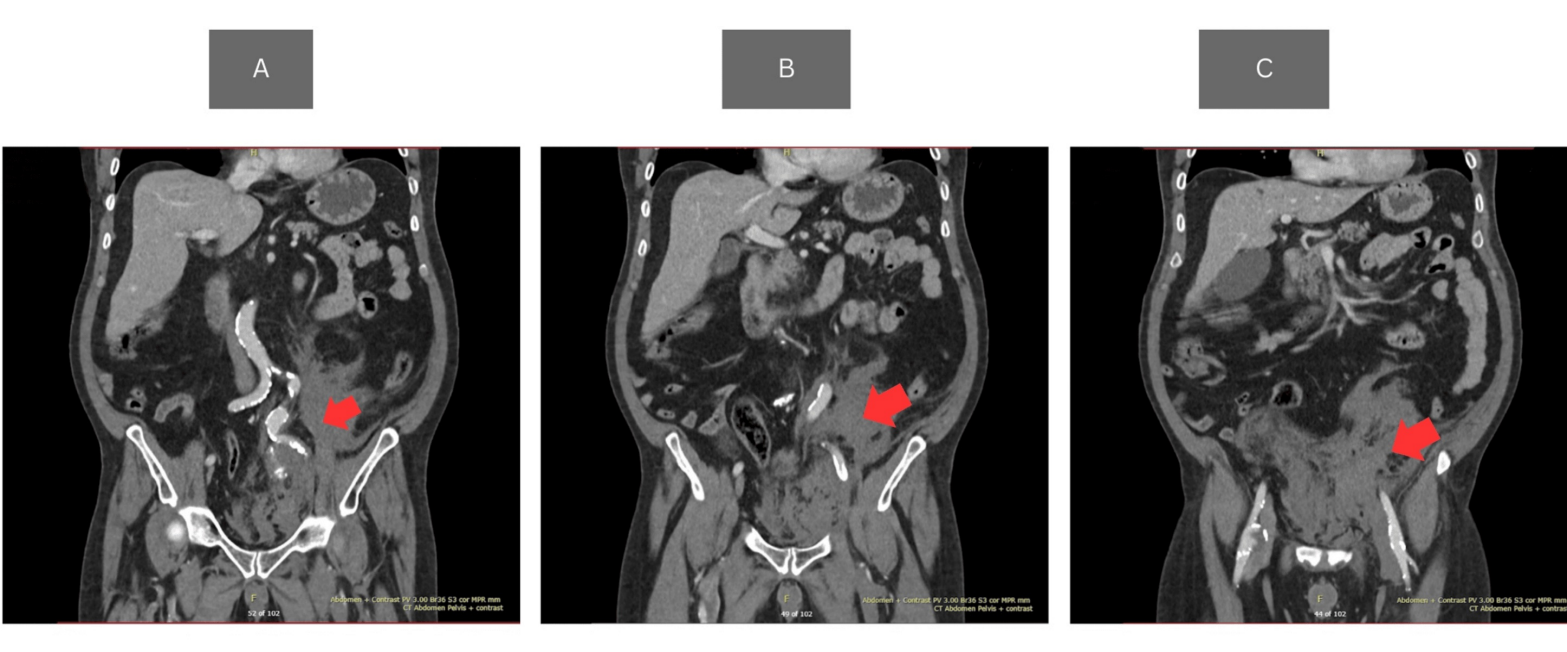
CT abdomen with contrast enhanced (A) Abrupt interruption of the vessel (red arrow); (B) progressive collapse and irregularity of ruptured artery segment (red arrow); (C) absence of distal internal iliac artery flow beyond the rupture site (red arrow) CT: computed tomography
